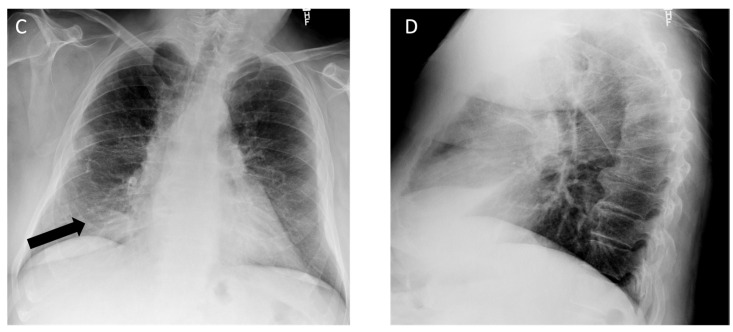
A 56-year-old male with a history of grinding aluminum for 5 years diagnosed with desquamative interstitial pneumonia (DIP) on surgical lung biopsy. There was a 10 pack year smoking history, but it was remote to the presentation, and the DIP was attributed to occupational exposure. (A) Axial computed tomography of the upper lung zone reveals emphysema (white circle), and the (B) basal lung zone demonstrates extensive ground glass opacity (arrows) with subtle small cysts or lucencies on close inspection; (C) posteroanterior and (D) lateral view chest radiographs 6 months later show persistent basilar opacities (black arrow).
